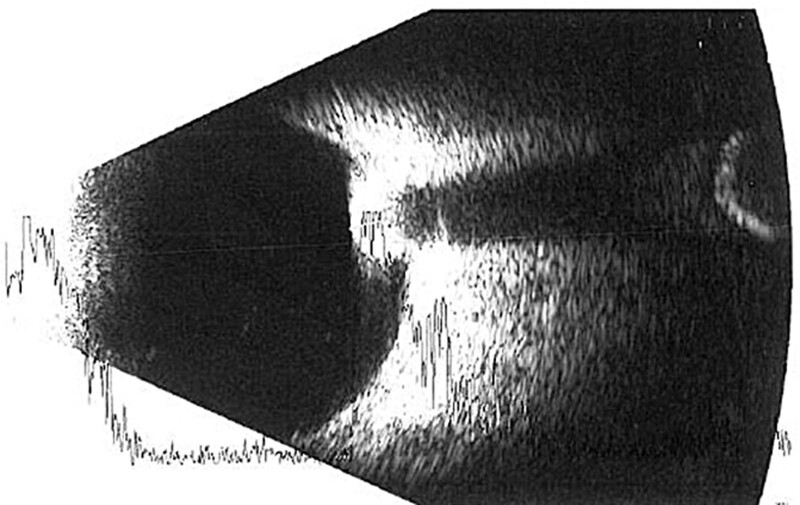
Left eye ultrasonography.
